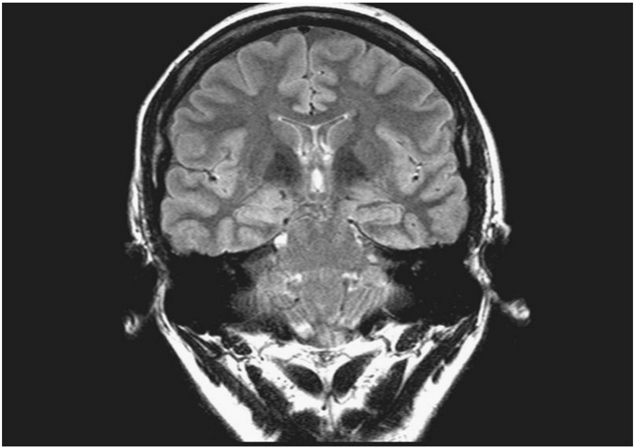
Brain MRI shows symmetrical normal-sized hippocampi.
